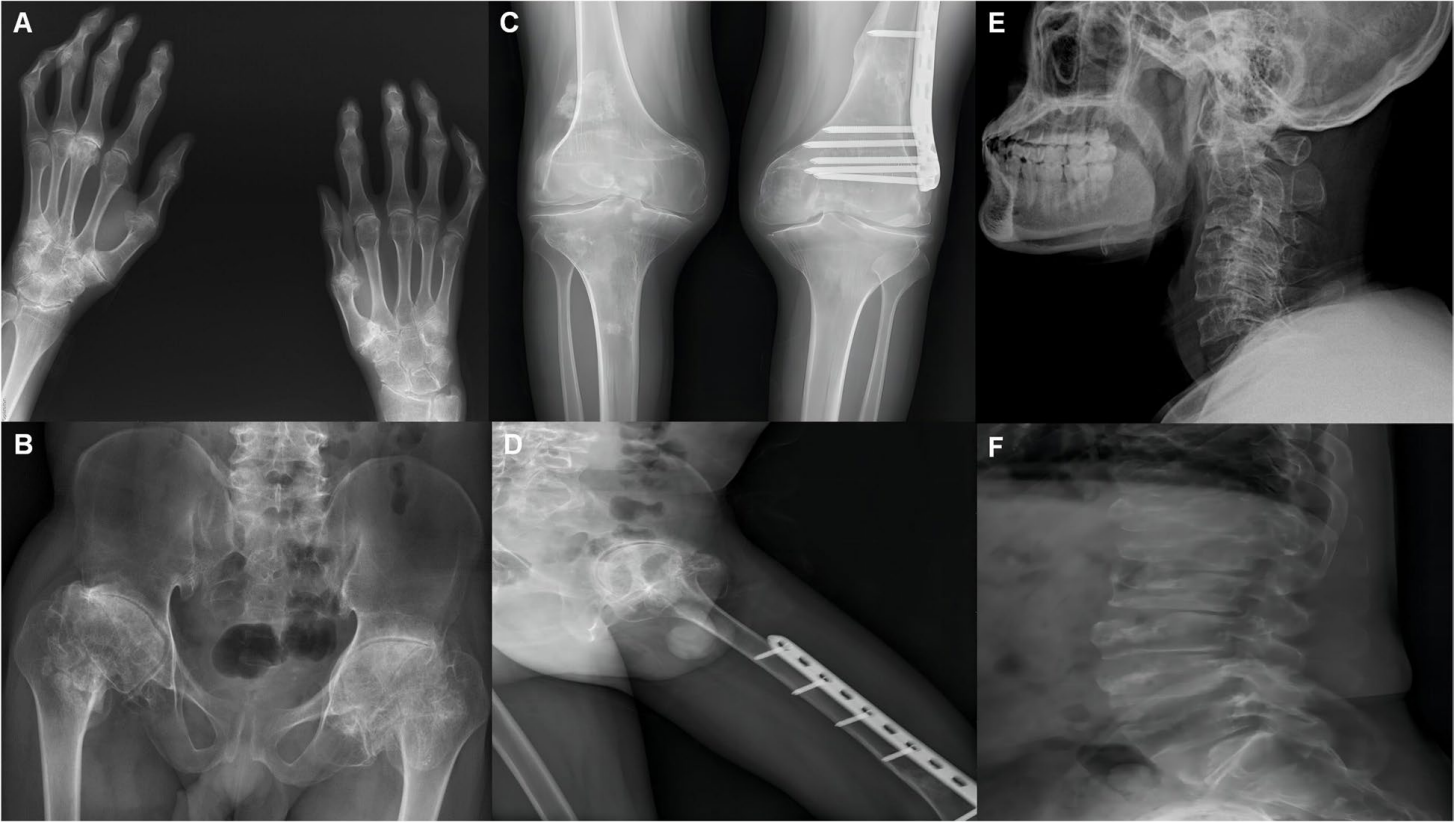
Hand and pelvis x-rays (a, b) showed increased light transmittance, narrowing of the joint space, hyperostosis and sclerosis of the subarticular surface, and bony enlargement. Bilateral femoral neck shortening, discontinuous calve line. The left femur (c, d) was fixed in place, the bone structure of the lower femur was disordered, and callus formed. Cervical and lumbar (e, f) decreased bone mineral density, vertebral bodies were compressed and flattened to varying degrees, marginal osteophytes were formed, and endplates were irregular
